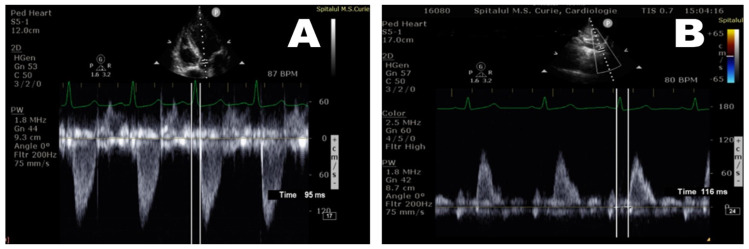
Measurement of ΔT2 (T2-T1 = 116 − 95 = 21 ms) in the descending aorta—echocardiography 5C apical window (A) and subcostal view (B).
